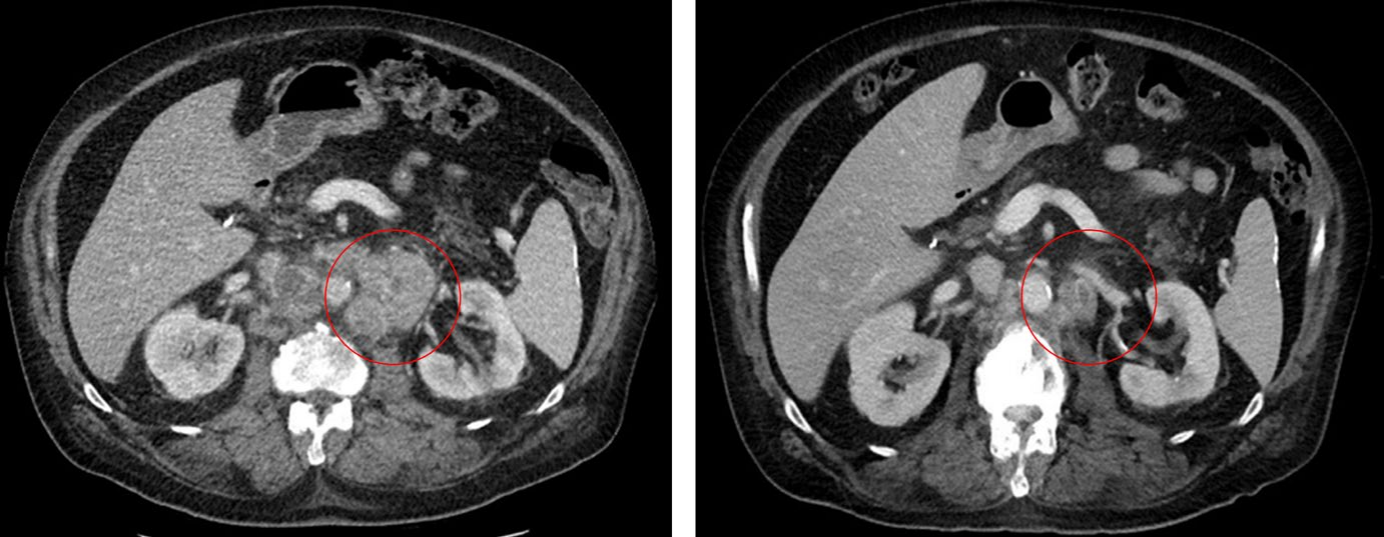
Radiograph showing partial response from patient 3. Representative CT scans of a patient with a partial imaging response (PR). CT scan 3 months out (right) shows substantially decreased size of bulky retroperitoneal lymphadenopathy. His PSA remained >90% below baseline and he remained on treatment at study end date, having received 11 cycles thus far
